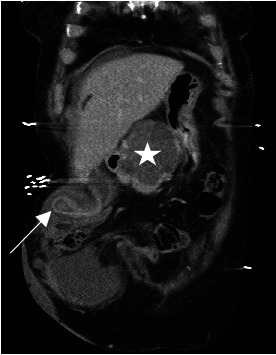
Computed tomography scan of gallbladder herniation (arrow) and large complex cystic head of pancreas lesion (star) in coronal plane.
